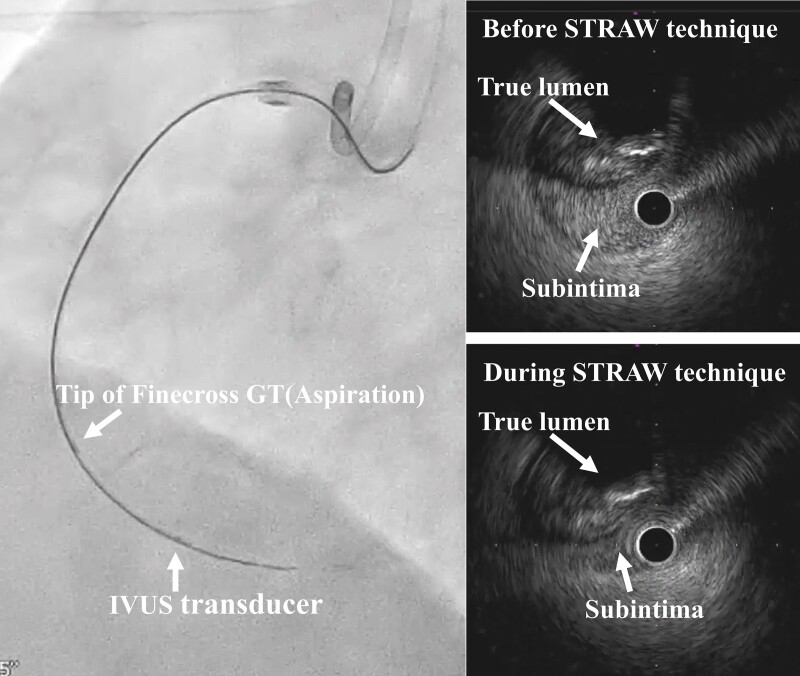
Intravascular ultrasound images before and during the subintimal transcatheter withdrawal technique. IVUS, intravascular ultrasound; STRAW, subintimal transcatheter withdrawal.
